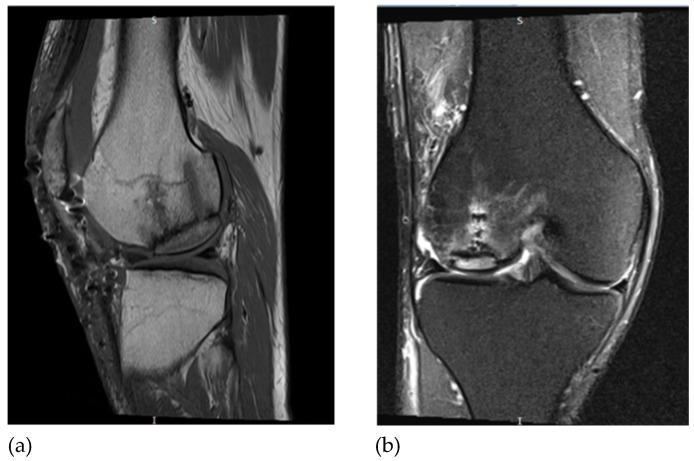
Postoperative knee MRI; (a) sagittal view; (b) coronal view.
